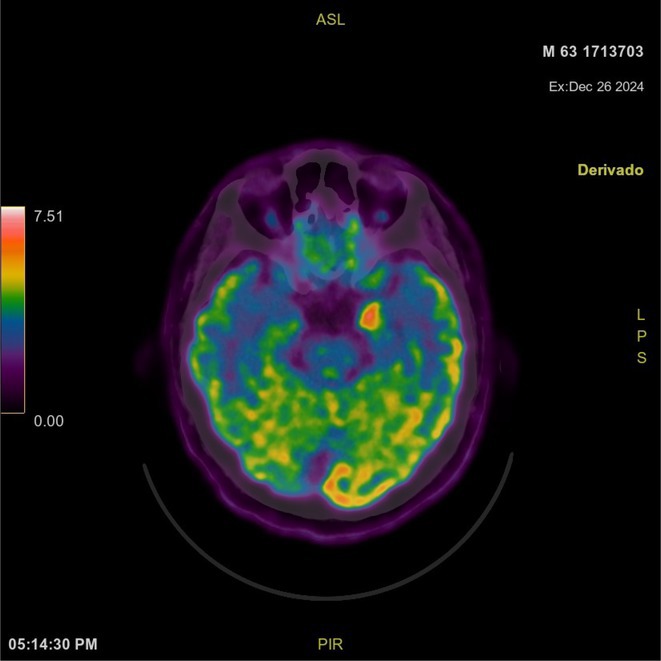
FIGURE 2 Brain PET/CT scan revealing enhancement in the medial area of the left temporal lobe.
